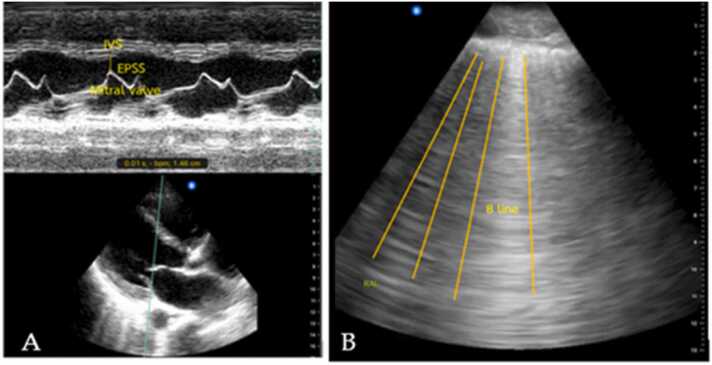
Representative of ultrasound image findings for cardiogenic shock; decrease left ventricle contraction (A) and multiple B-lines (B).
